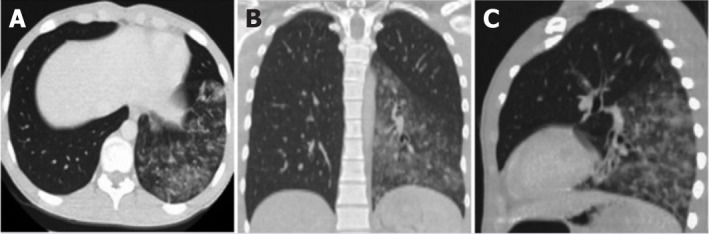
Axial, coronal and sagittal reformatted thoracic computed tomography images at parenchymal window showing ground-glass opacities and areas of consolidation, followed widely in the lower lobe of the left lung, compatible with pulmonary contusion and alveolar hemorrhage.
